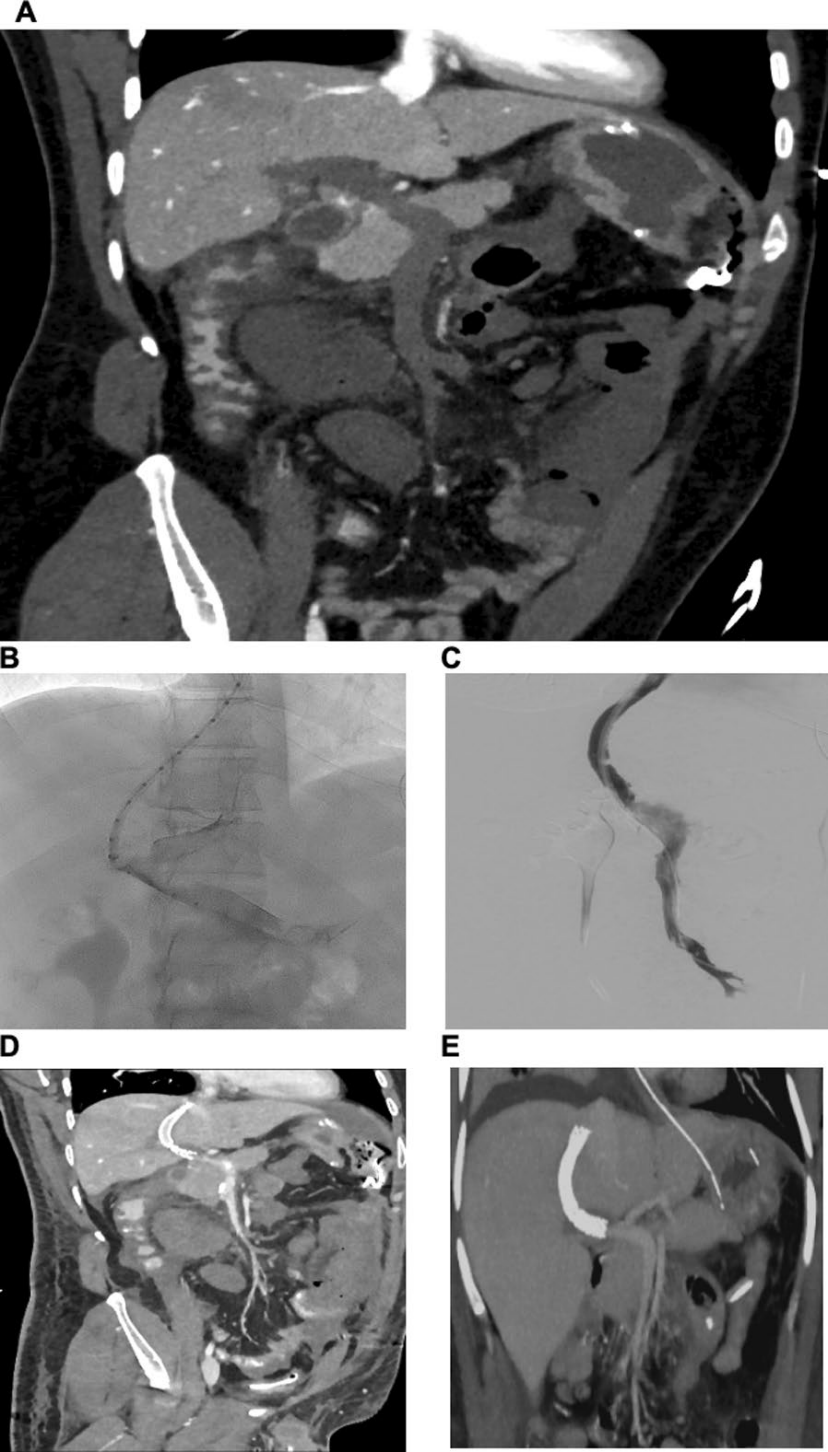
Radiographic images of portomesenteric thrombosis and TIPS insertion. A Coronal computed tomography image, showing extended portomesenteric thrombosis pre-TIPS. B Angiography showing portal thrombosis and insertion of measuring catheter. C Angiography showing TIPS insertion, mild residual thrombosis after thrombaspiration, and local lysis. D Coronal computed tomography (CT) post TIPS and local lysis. E Coronal computed tomography (CT) post TIPS CT and post resection of small intestine, restituted portomesenteric flow with no signs of relevant residual thrombosis
